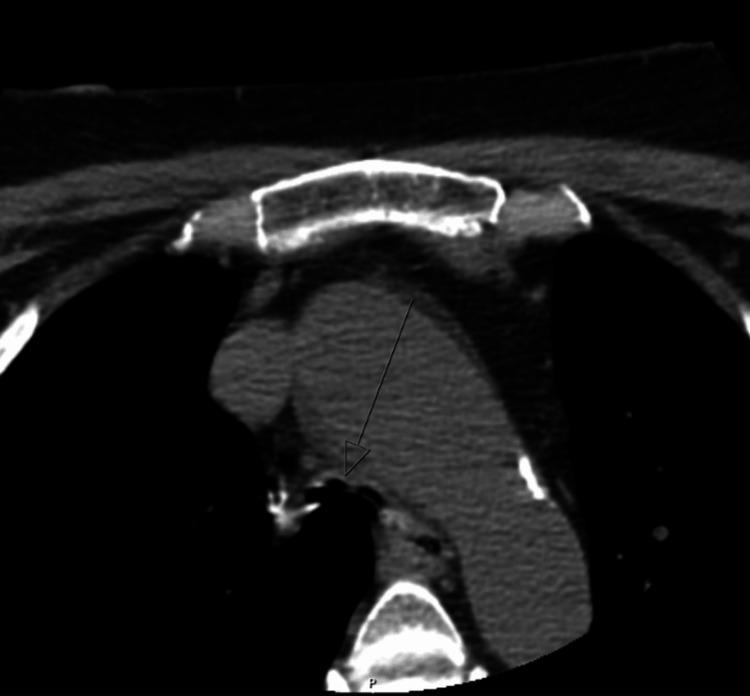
CT image of soft tissue neck without contrast (axial view) The image is showing stenosis of the trachea.
